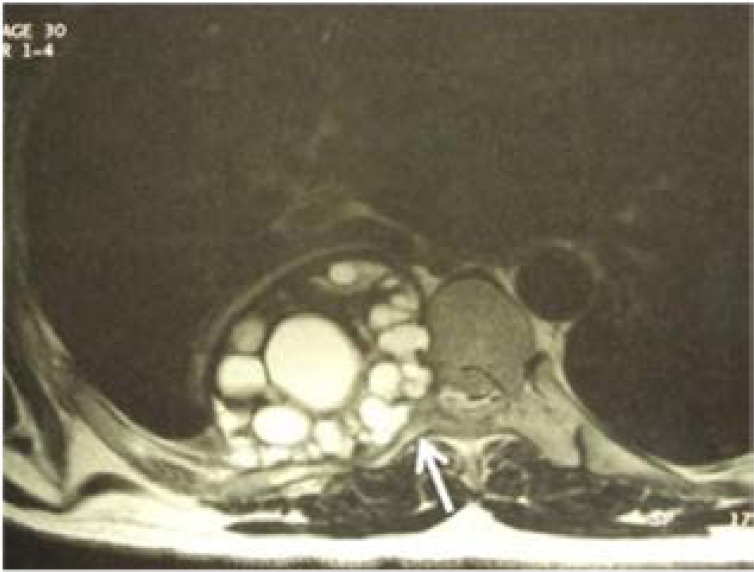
Transverse section thoracic MRI showing right multivesicular lesion of the costovertebral gutter, with involvement of the posterior arch of the 7th rib and the 7th dorsal vertebra (arrow), presenting a foraminal and endocanalar extension.
